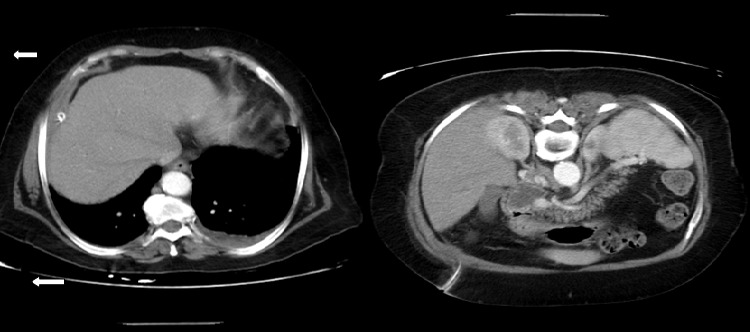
Abdominal CT showing drain (white arrow) with resolution of the biloma
